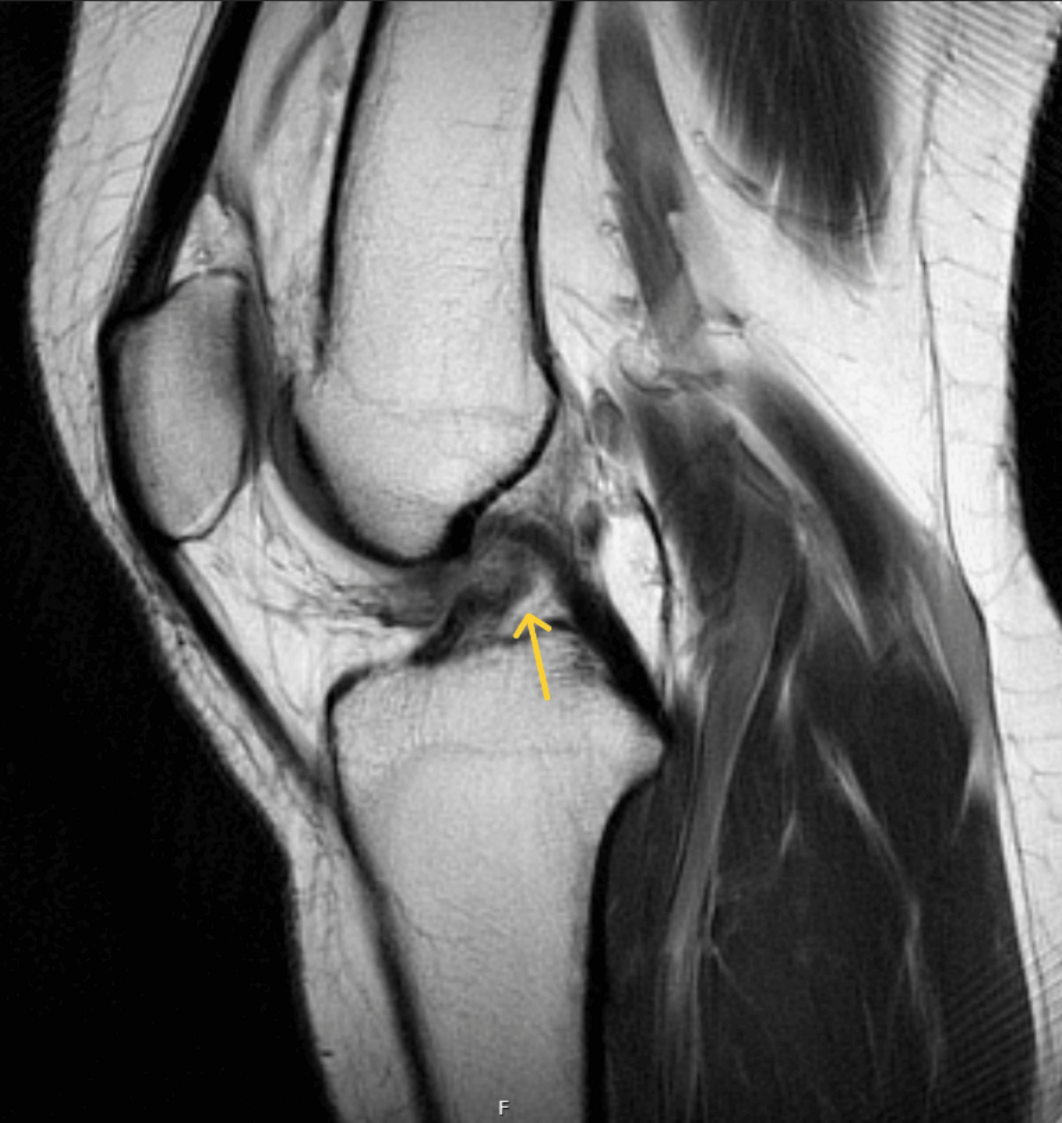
Sagittal proton density MRI of the right knee demonstrating an ACL tear (yellow arrow) ACL: anterior cruciate ligament
